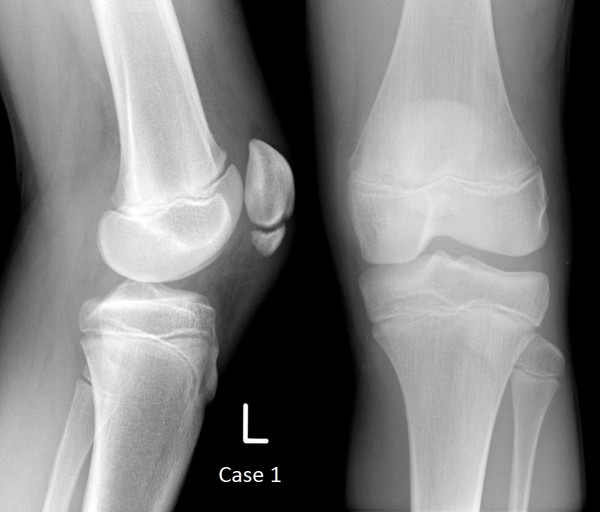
Plain film radiographs of Case 1 at presentation.
